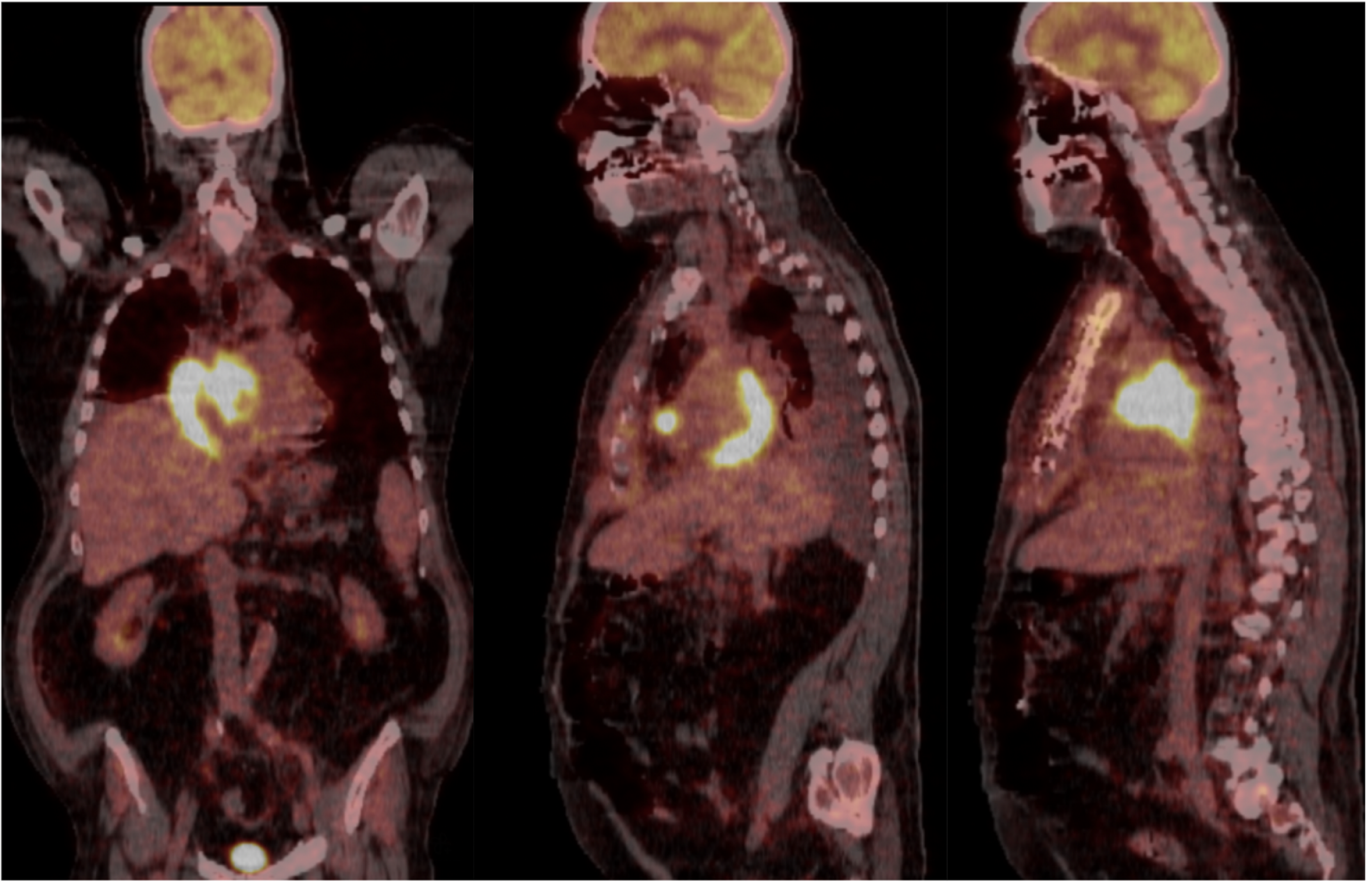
Whole-body positron emission tomography/computed tomography (PET/CT) in coronal and sagittal views showing pronounced uptake of fluorine-18 fluorodeoxyglucose in the right atrium, with no other metabolically active lesions.
